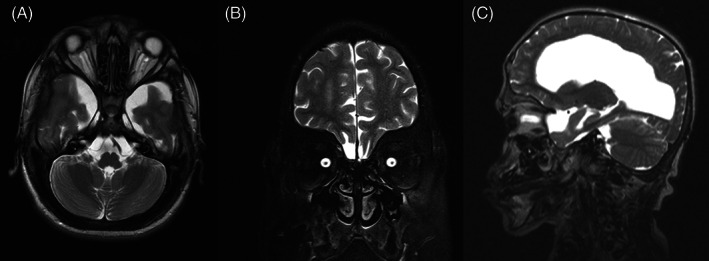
T2‐fat saturation MRI for patient 5 showing enlarged perioptic subarachnoid space with reduced optic nerve thickness. (A) Axial section; (B) coronal section; (C) sagittal section
